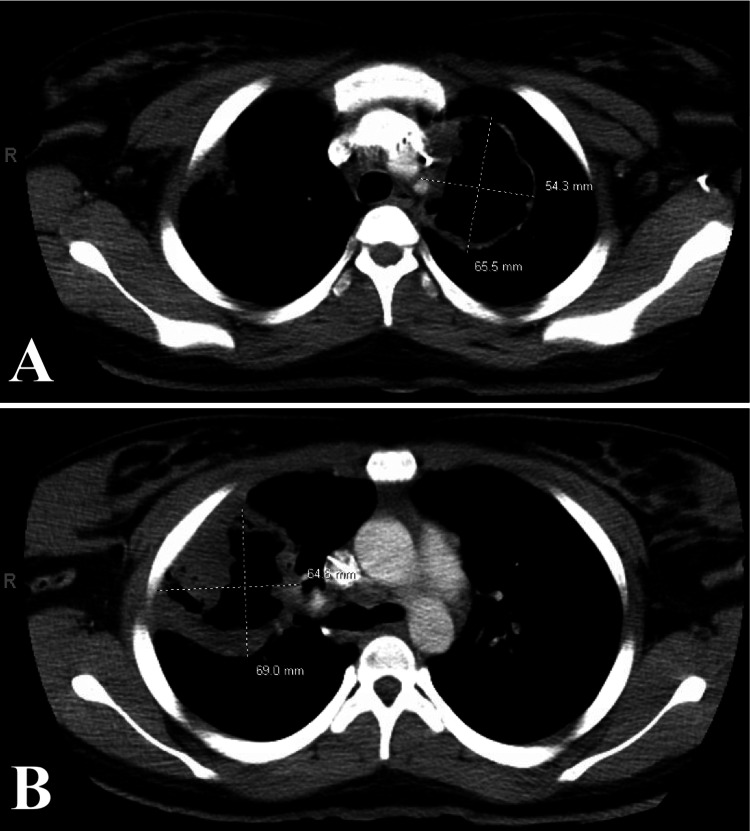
CT chest with cavitating lesions in the left (A) and right (B) lungs Cross-sectional cavity measurements displayed in A and B CT: computed tomography
